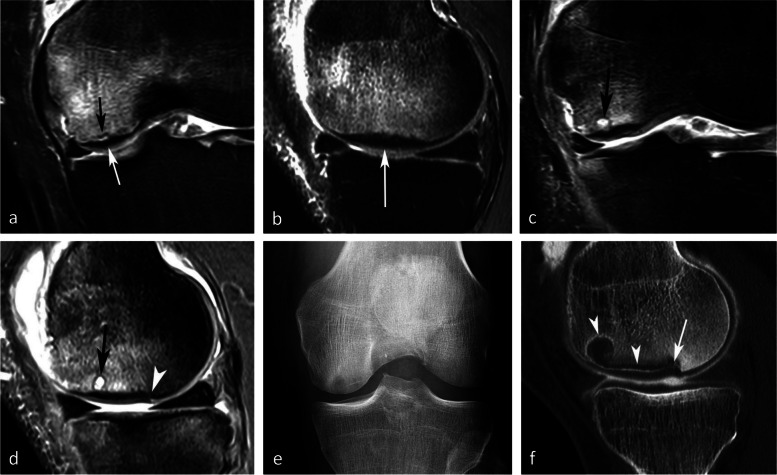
Example of a pejorative evolution of a SIF to a SIF-ON. a, b At the initial stage, the BME-like high intensity on fat-suppressed T2-weighted MR image and a small fissure line (black arrow in a) are suggestive of a SIF. However, the subchondral bone adjacent to the cartilage shows a thick and extensive low signal-intensity area (white arrows in a and b). c, d Five months later (c, d) the marrow abnormalities persist, with the appearance of small geode-like images (arrows) and a small break in the subchondral bone plate (arrowhead in d). e, f Three months later, radiograph (e) still shows heterogeneous density of the lower condylar pole, and CT arthrography (f) shows a subchondral geode and bone resorption (arrowheads), associated with a focal collapse of the subchondral bone surface (arrow)
